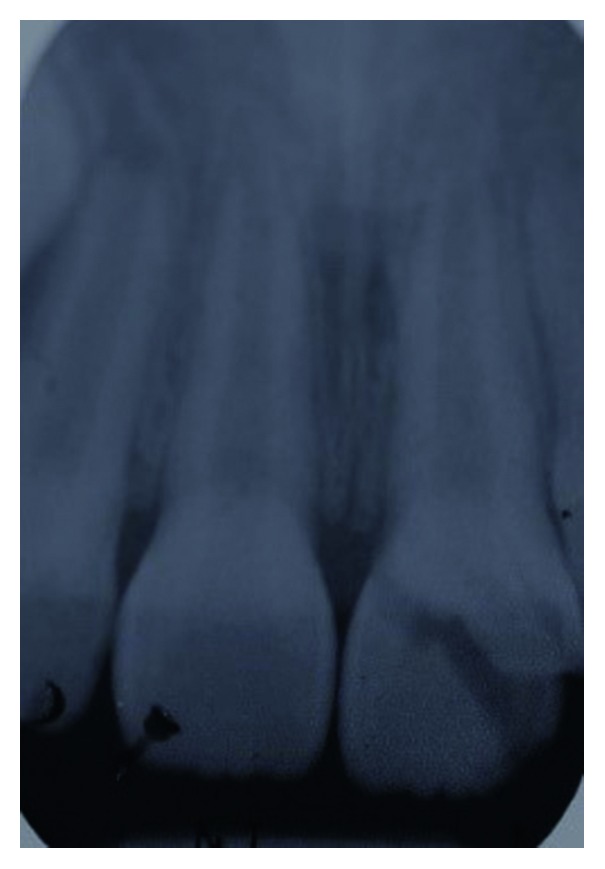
Periapical radiograph of tooth 21 showing the extent of the crown-root fracture.
